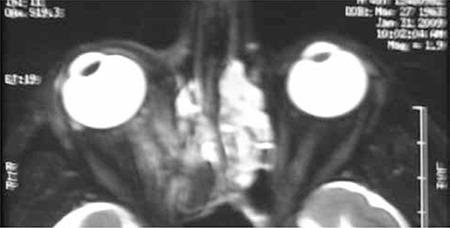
Case 1, MRI revealed bone defect on the right medial orbit wall; the medial rectus was deviated medially and was adherent to this defect. There was no apparent connection between the proximal and distal segments of the muscle
